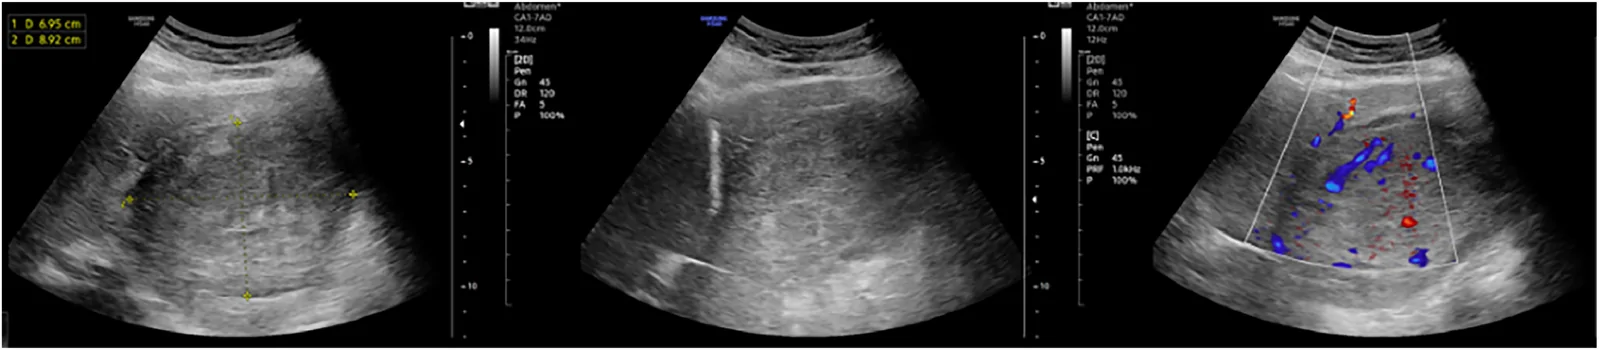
Ultrasound evaluation before biopsy and embolization. (A) B-mode ultrasound demonstrates a large heterogeneous left hepatic lobe mass with internal complexity compatible with hemorrhagic and necrotic components. (B) Ultrasound image obtained during biopsy planning demonstrates the selected needle trajectory through a relatively avascular portion of the lesion. (C) Color Doppler ultrasound demonstrates prominent internal vascularity, supporting the hypervascular nature of the mass. These findings guided selection of a safer biopsy trajectory while minimizing traversal of vascular tumor components.
